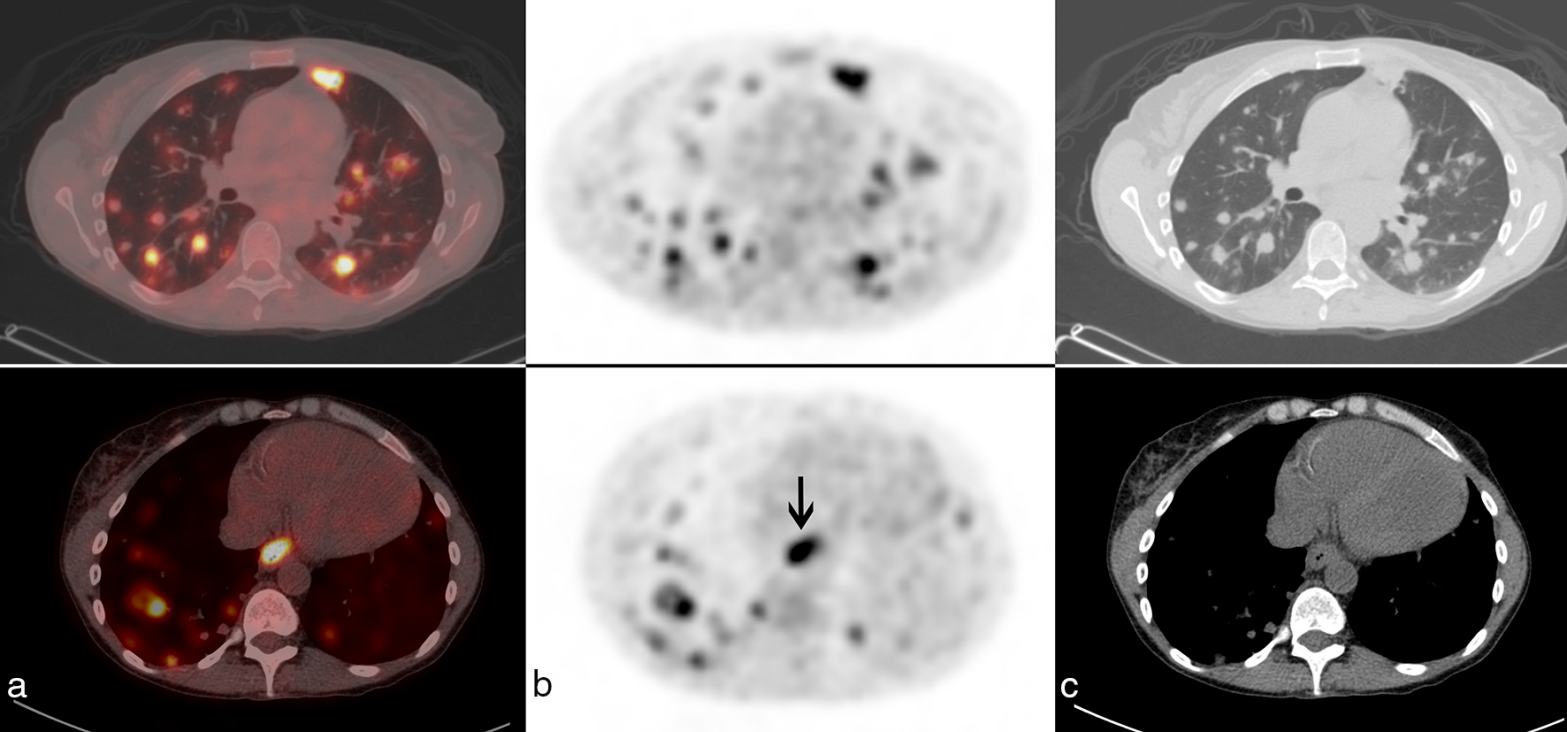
Transaxial (a) positron emission tomography/CT fusion, (b) positron emission tomography and (c) CT images showing the innumerable ^18^F-fludeoxyglucose-avid lung nodules, which were irregular but well defined on CT images, and a distal oesophageal focus of intense ^18^F-fludeoxyglucose uptake [arrow in (b)].
